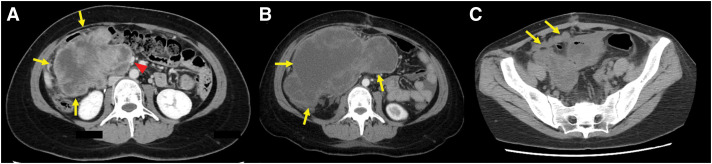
(A) Contrast-enhanced computed tomography indicated a large, irregular mass (arrows) on the right side of the abdominal cavity. The mass was extensively bordered by the narrow superior mesenteric vein (arrowhead). (B) Contrast-enhanced computed tomography revealed the markedly enlarged tumor (arrows). (C) A follow-up CT on postoperative day 14 revealed multiple peritoneal nodules (arrows).
